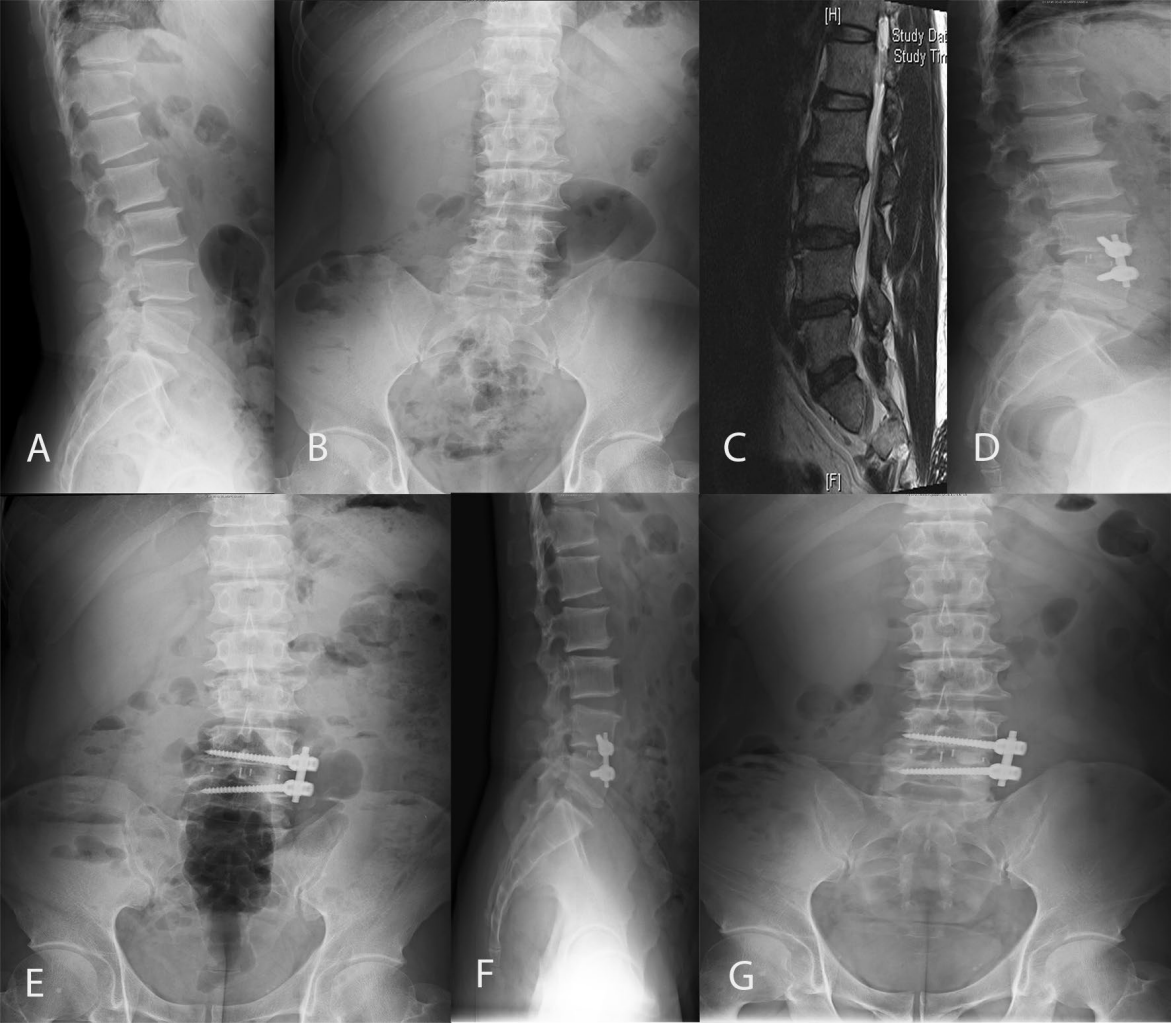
A patient was diagnosed with degenerative spondylolisthesis and lumbar spinal stenosis at L4-5 level, as shown on lumbar spine lateral view (A), lumbar antero-posterior view (B), and T2-weighted MR image (C). He received oblique lumbar interbody fusion (OLIF) at L4-L5 with lumbar spine lateral view (D) and lumbar antero-posterior view images (E) taken immediately after the surgery. Follow-up images (F,G) were taken at the last follow-up.
